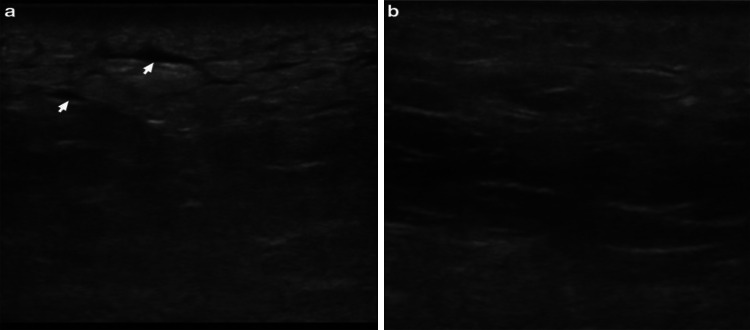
Ultrasonography of left gluteal soft tissue with a necrotizing fasciitis four days post-hospitalization and b 8 days post-hospitalization using a linear probe. These images show the progression of the sonographic appearance of necrotizing fasciitis, initially with an accumulation of fluid in the subcutaneous tissue (arrows), giving it a “cobblestone” appearance and a progressive return to the normal architecture of the subcutaneous tissue over time. Source: Magalhaes et al. with permission
